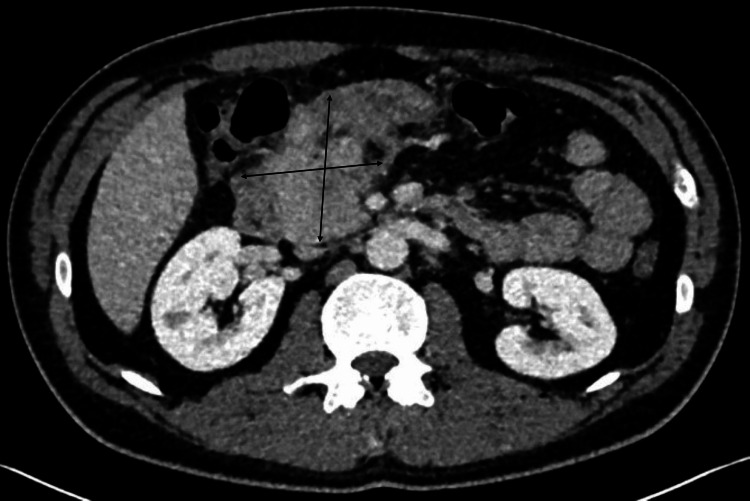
CT abdomen with contrast CT abdomen with contrast revealing a pancreatic head mass.
